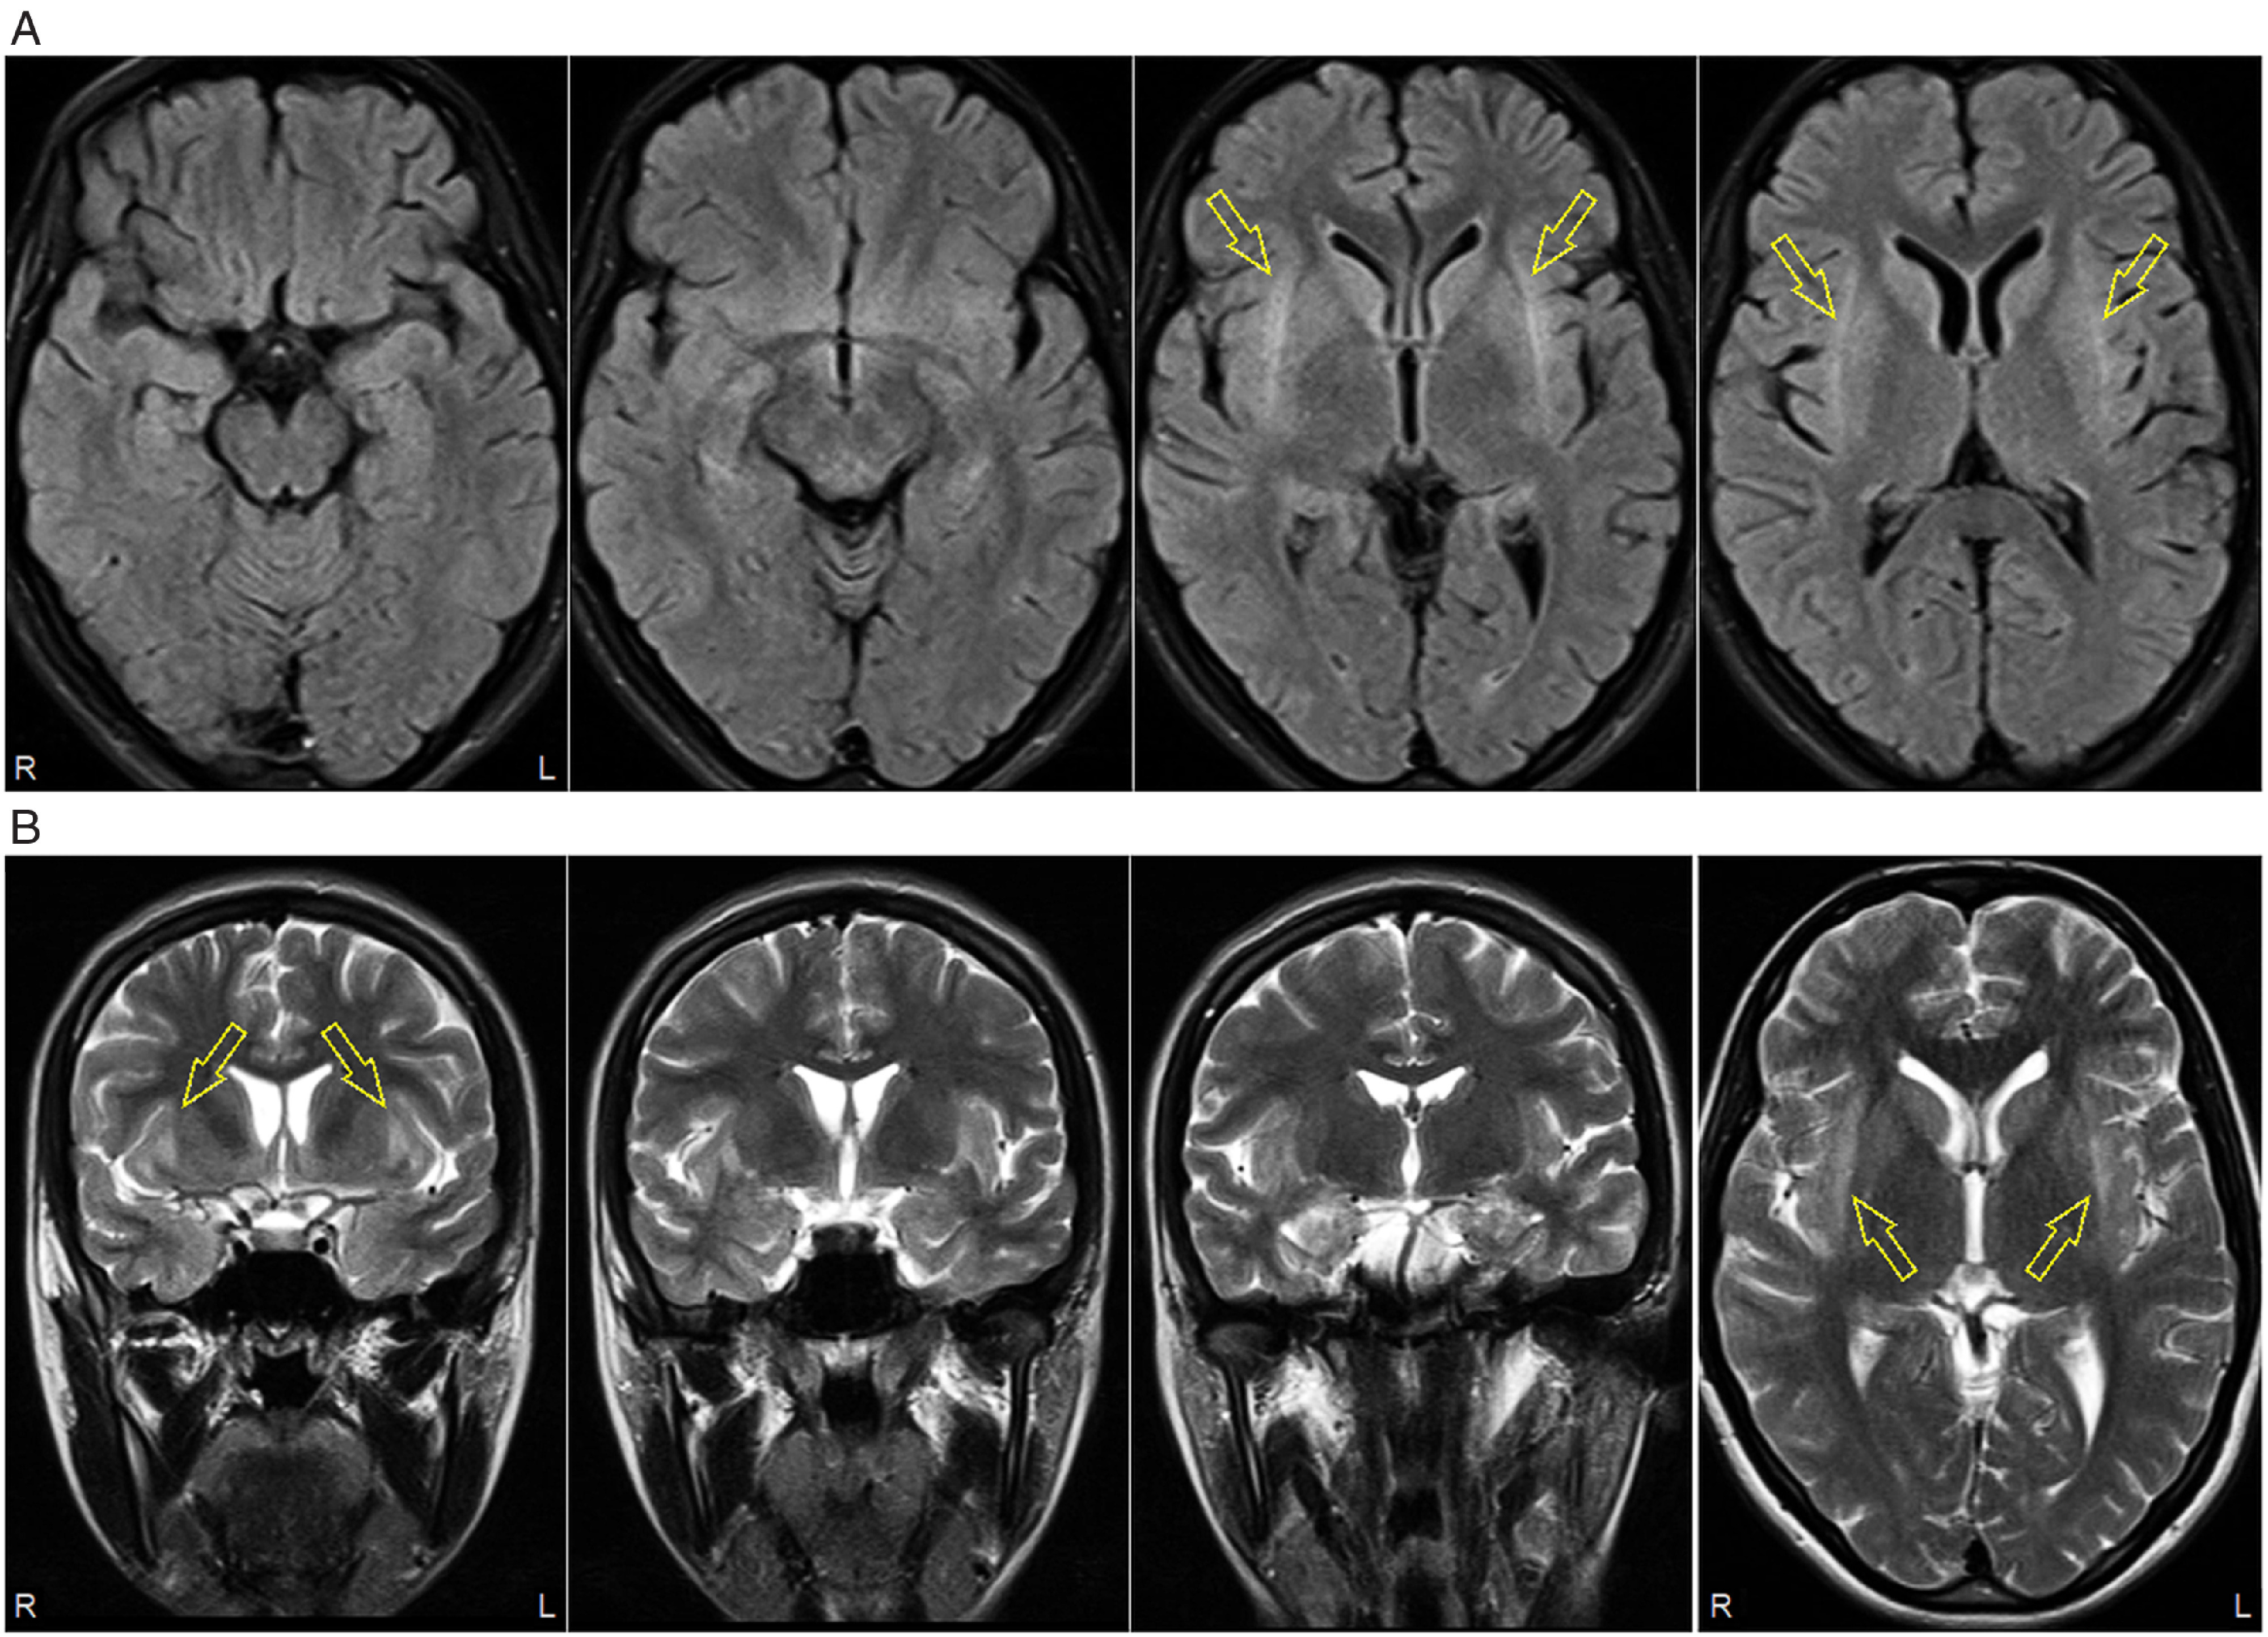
Brain MRI 14 days after initial admission. (A) Axial fluid-attenuated inversion recovery (FLAIR) sequence showing bilateral claustrum hyperintensities (arrows); mesial temporal structures unremarkable. (B) Coronal and axial (far right panel) T2-weighted sequences showing bilateral claustrum hyperintensities extending to external and extreme capsules, with questionable involvement of adjacent insular cortices (arrows).
